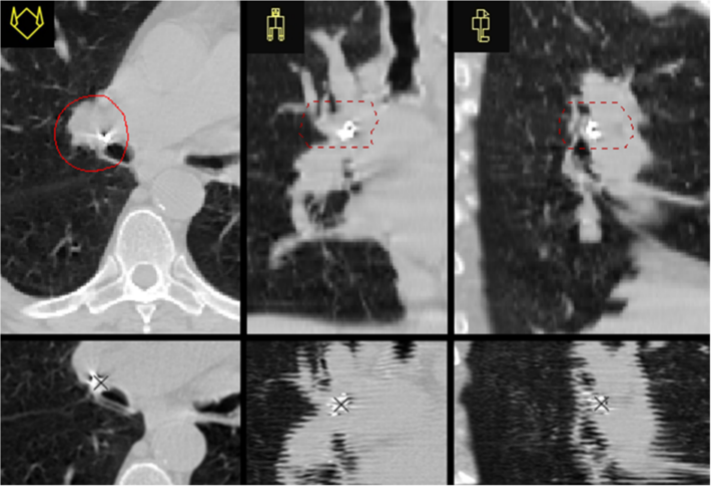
Treatment planning computer tomography of carcinoma in situ. Planning CT shows the position of the Visicoil radiographic marker in transversal, sagittal and coronal sections with respect to the right upper lobe main bronchus and demonstrates the ellipsoidal PTV with respect to the Visicoil radiographic marker and the left upper lobe main bronchus in transversal, sagittal and coronal slices. Upper row: fast helical scan of planning CT; lower row: slow helical high resolution scan acquired during verification procedure. Whereas in fast scanning mode almost no motion of radiographic marker can be seen, breathing motion becomes apparent in slow helical scanning mode. For verification of correct treatment position therefore the latter scanning mode was chosen. Radiographic marker movement center was found by means of a 3D-cursor (cross).
